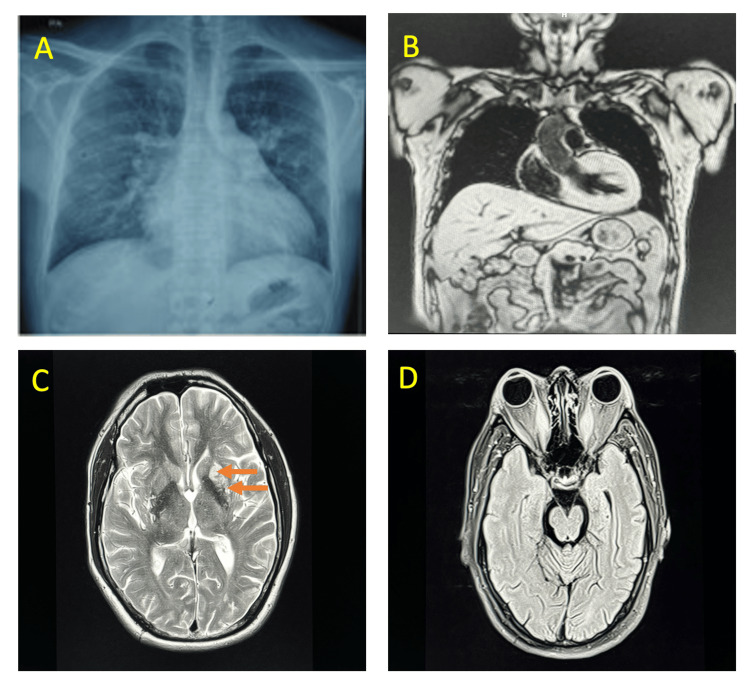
Investigations of the patient. (A) Chest X-ray posteroanterior view revealing cardiomegaly. (B) Cardiac MRI study revealing asymmetrical hypertrophic cardiomyopathy. (C) MRI of the brain, axial cuts (arrows) showing chronic lacunar infarcts in left centrum semiovale and corona radiate. (D) MRI orbits showing enlargement of bilateral recti muscles with resultant bilateral proptosis.
